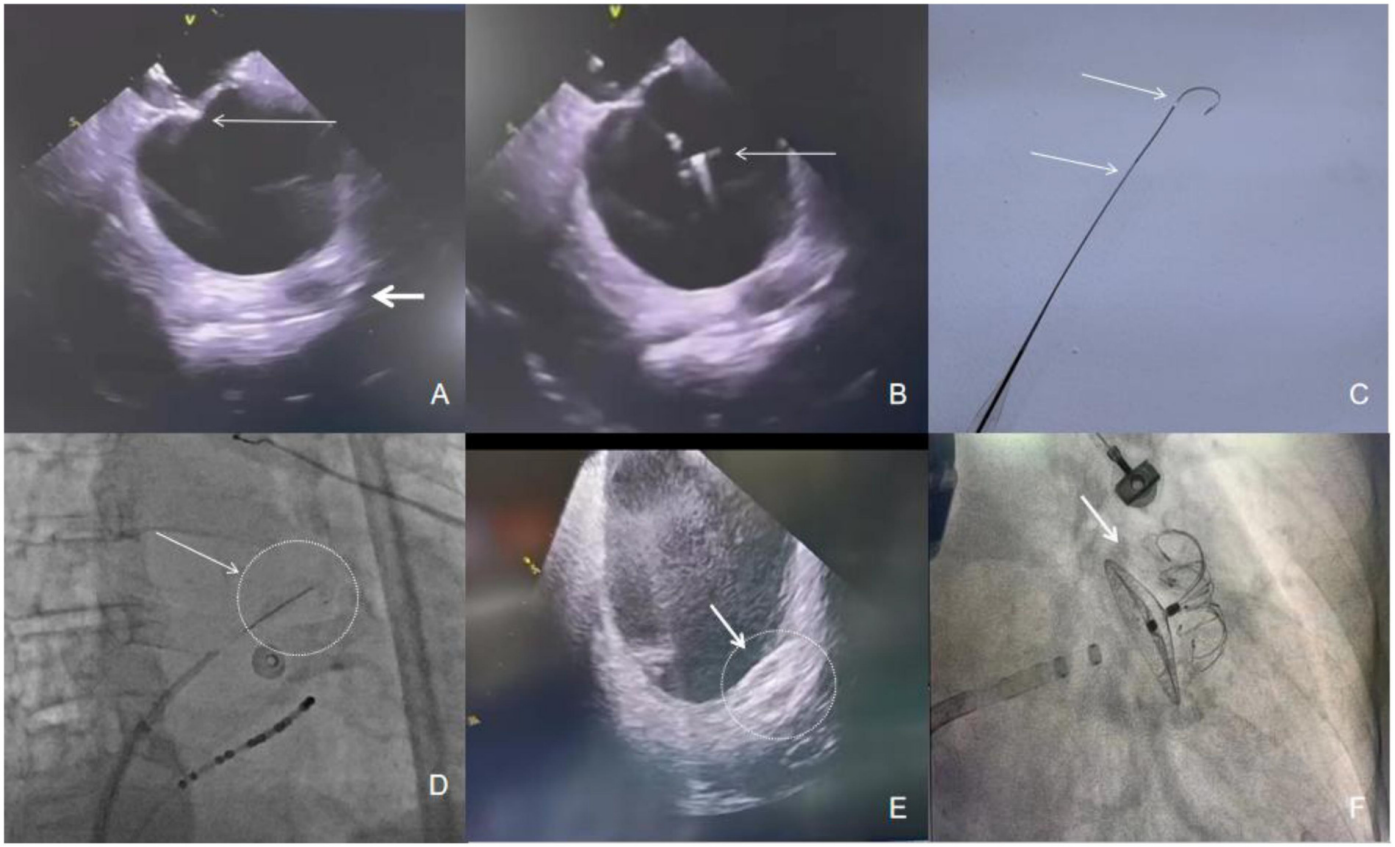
(Panel A) Small arrow: ICE shows “tenting” in atrial septal, big arrow: LAA, which indicates the posterior position of the atrial septal under ICE. (Panel B) Small arrow: the head of the Accusafe guidewire in the left atrium. (Panel C) Shape of guidewire and radiopaque period. (Panel D) Arrow and Circle: The tip and proximal period of the guidewire are radiopaque to indicate the position of the wire under x-ray. (Panel E) Arrow and Circle: ICE shows Lamax device was firmly planted in LAA. (Panel F) Arrow: Final shape of Lamax device in LAA by fluoroscopy.
